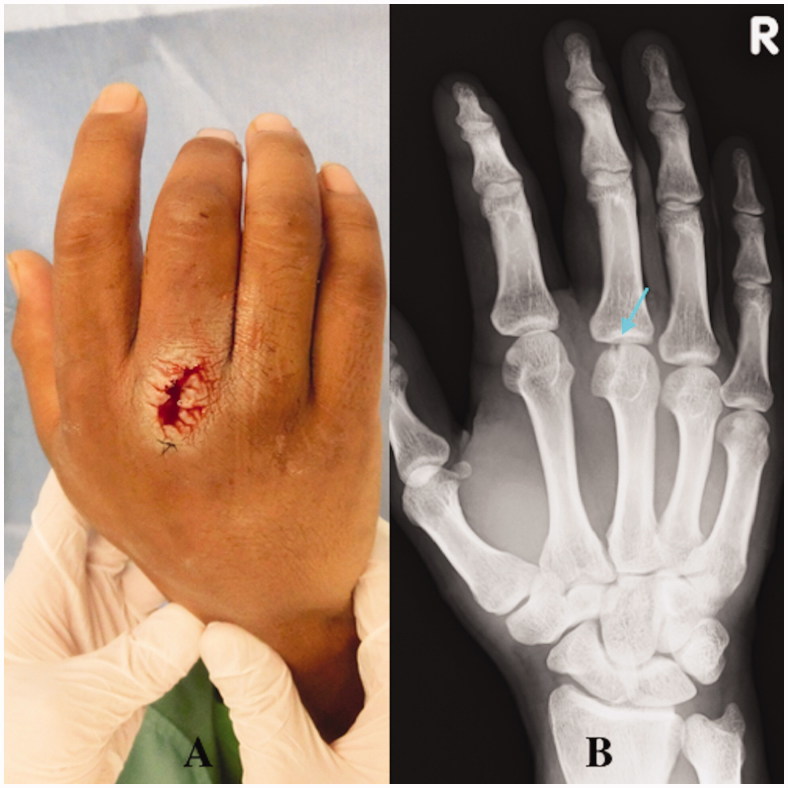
Case 2. (A) Noticeable swelling, erythema, and pus-like discharge on the dorsum of the third MCP joint. (B) The arrow shows a visible tooth sign in an oblique radiographic view.
